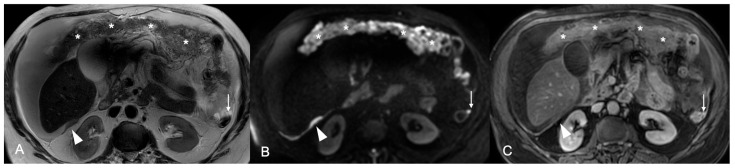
Axial MR images of the upper abdomen. (A) T2W image shows diffuse involvement of the greater omentum with “omental cake” pattern (asterisks). There is also a 15 mm nodule in the Morisson space (arrowhead) and a 5 mm nodule in the left paracolic gutter (arrow). Peritoneal deposits demonstrate high signal intensity on the b 1000 DWI image (B), indicative of restricted diffusion, and enhancement on the T1W post-contrast sequence with fat saturation (C).
